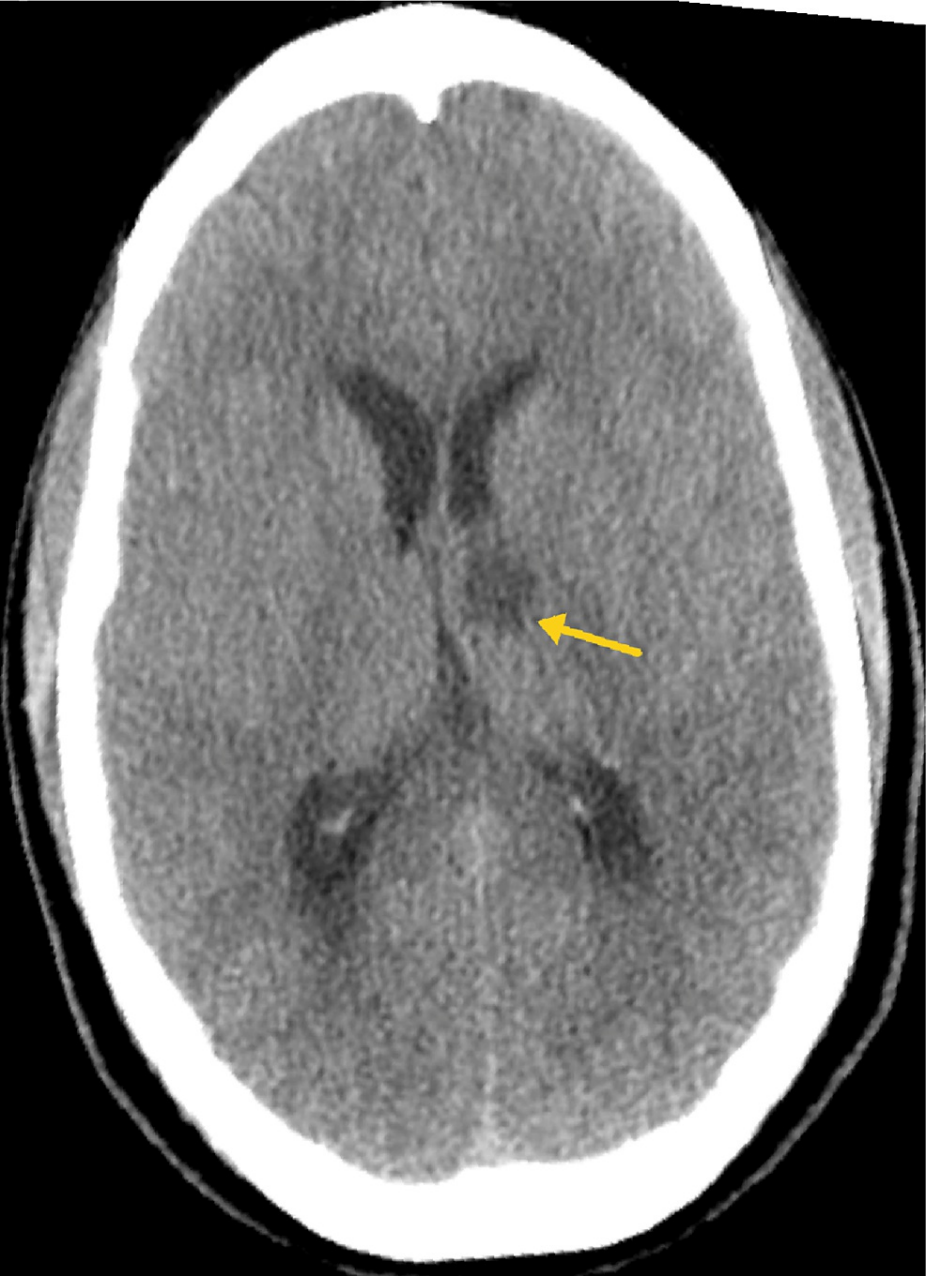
Representative axial computed tomographic (CT) image through the level of the basal ganglia demonstrates new hypodensity in the anterior left thalamus concerning for ischemic infarct.
